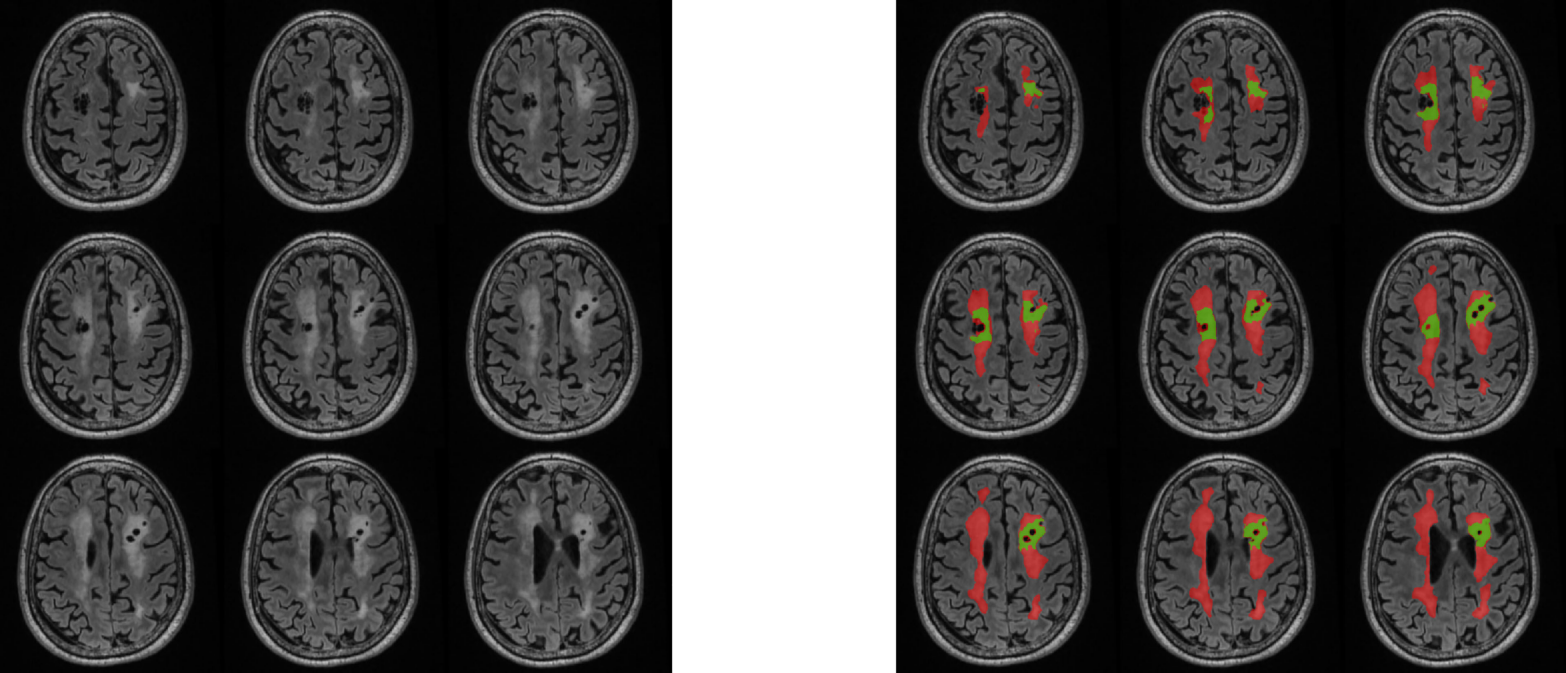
Visual representation of WML analysis. Imaging inclusive of manual T2-FLAIR white matter lesion segmentations with RT-induced (red) and non-RT-induced (green) labeling. RT, radiation therapy.
